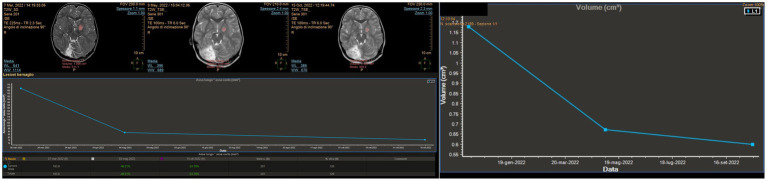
MITT Intellispace Portal imaging elaboration of magnetic resonance imaging shows a volumetric reduction of the known left thalamic tumor by more than 50% 7 months after surgery. The reduction of the proportion between the long and short axis is 54%.
